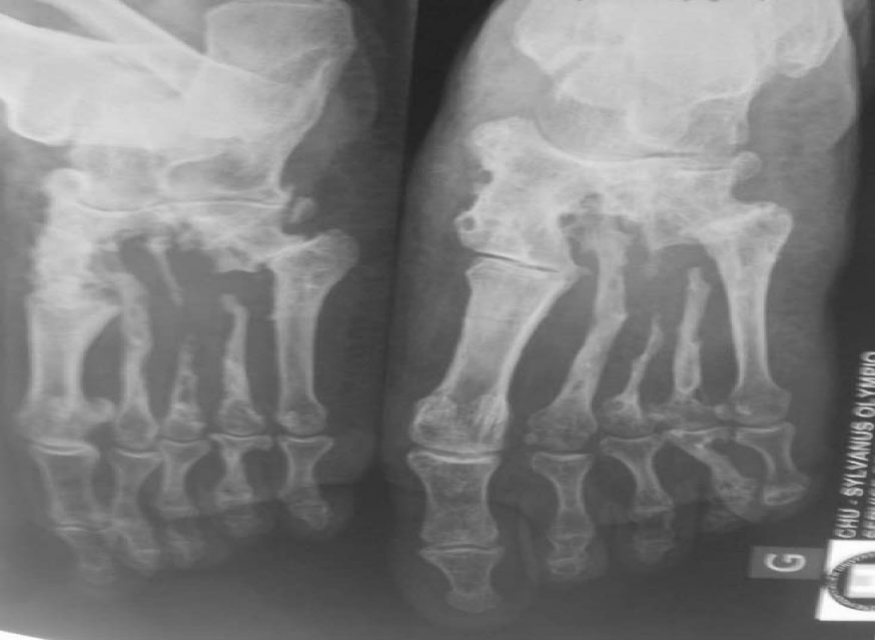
Radiography of feet showing bone destruction
